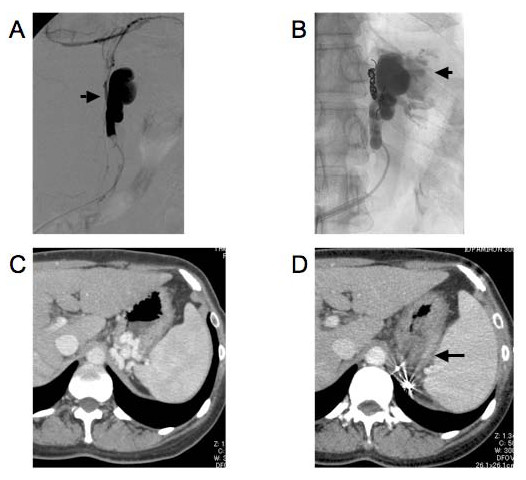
Balloon-occluded retrograde transvenous obliteration (B-RTO) performed on a 47-year-old woman with hepatitis C virus-related liver cirrhosis. A. A balloon catheter was inserted into the gastrorenal shunt. The gastric varices could not be clearly visualized on the venogram because the contrast medium had leaked into the retroperitoneal collateral veins (arrow). B. The retroperitoneal collateral veins were catheterized using a microcatheter and embolized using metallic coils. The gastric varices (arrow) could be clearly visualized on a venogram obtained after embolization of the retroperitoneal veins. C. A contrast-enhanced CT scan obtained before the B-RTO procedure showed gastric varices and gastrorenal shunts. D. A contrast-enhanced CT scan obtained 5 days after the first B-RTO procedure showed complete thrombosis of the gastric varices (arrowhead).
